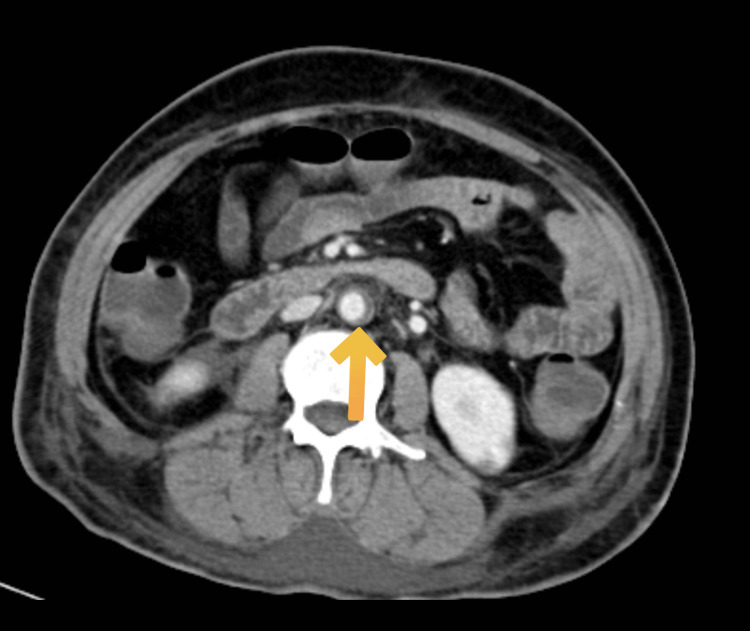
CECT abdomen (axial view) CECT: Contrast-enhanced computed tomography Eccentric partial atherosclerotic thrombus in the abdominal aorta (yellow arrow)
